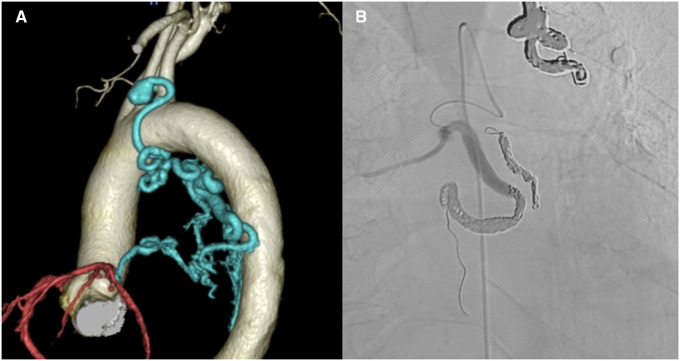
Preoperative Imaging. (A) CT angiography showing systemic-to-pulmonary artery feeders from the bronchial and left subclavian arteries. (B) Transarterial coil embolization of the systemic feeders.
